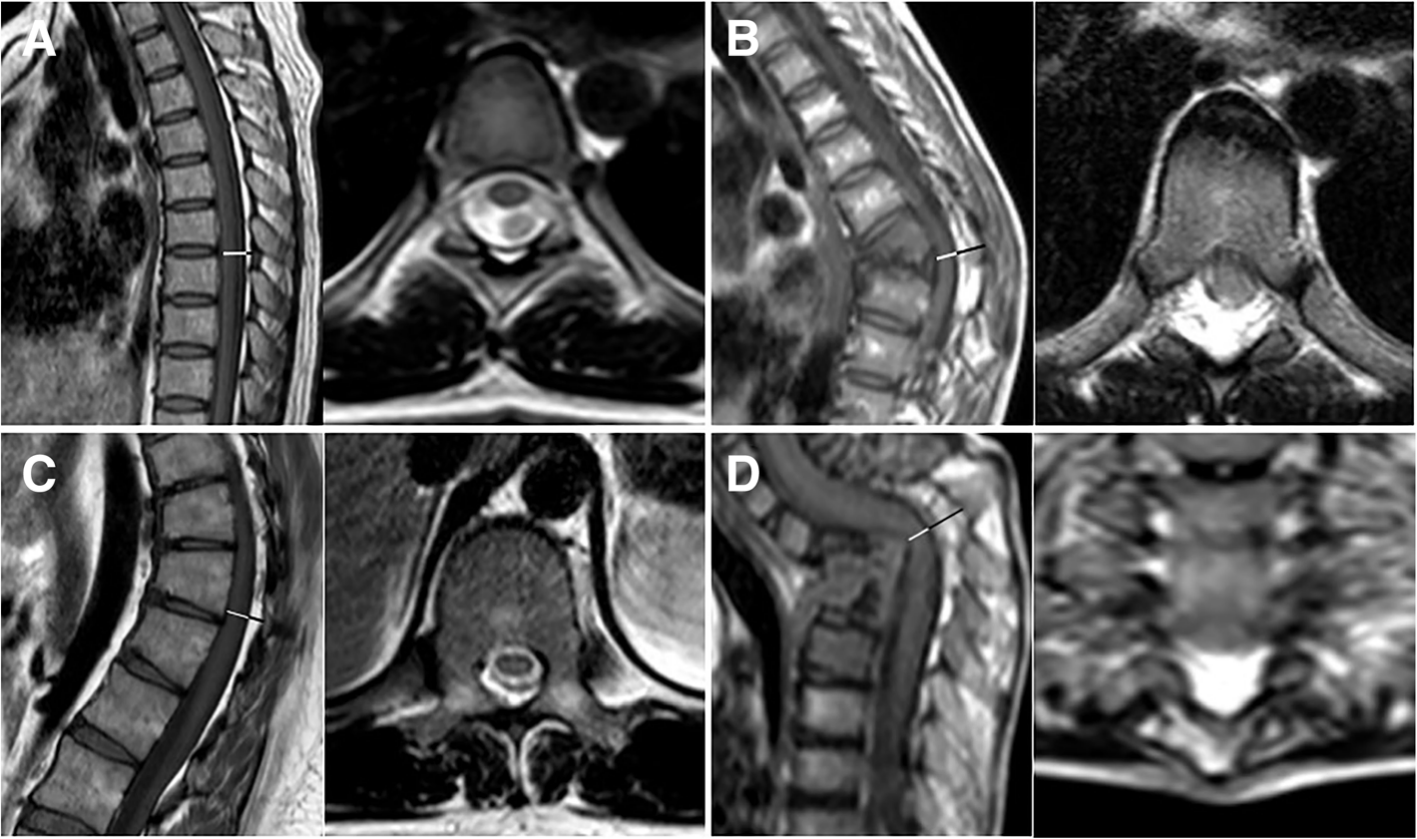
Sagittal and axial MR images of four different individuals included in this study. The measurements of the dural sac (DS) are shown in white, and those of the epidural fat (EF) are shown in black. a MRI of a control showing a normal amount of EF at T7 with an EF ratio (EFR_A_) of 0.22. b MRI of a patient with congenital kyphosis showing an increased amount of EF at T7 with an EFR_A_ of 0.57. c MRI of a patient with Scheuermann kyphosis showing an increased amount of EF at T7 with an EFR_A_ of 0.42. d MRI of a patient with tuberculotic kyphosis showing an increased amount of EF at T3 with an EFR_A_ of 0.64
